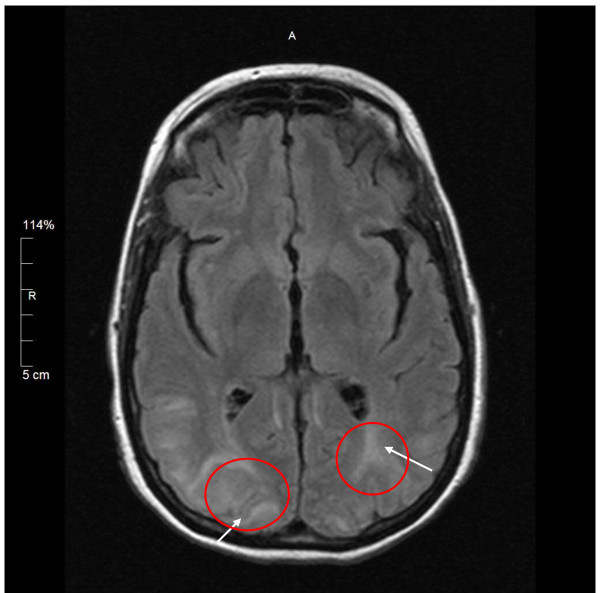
MRI findings in the brain of a patient with posterior reversible encephalopathy syndrome. Multifocal, T2-weighted, hyperintense lesions are present within the parasagittal posterior parietal lobe, both cerebellar hemispheres, and the occipital lobes bilaterally (arrows).
